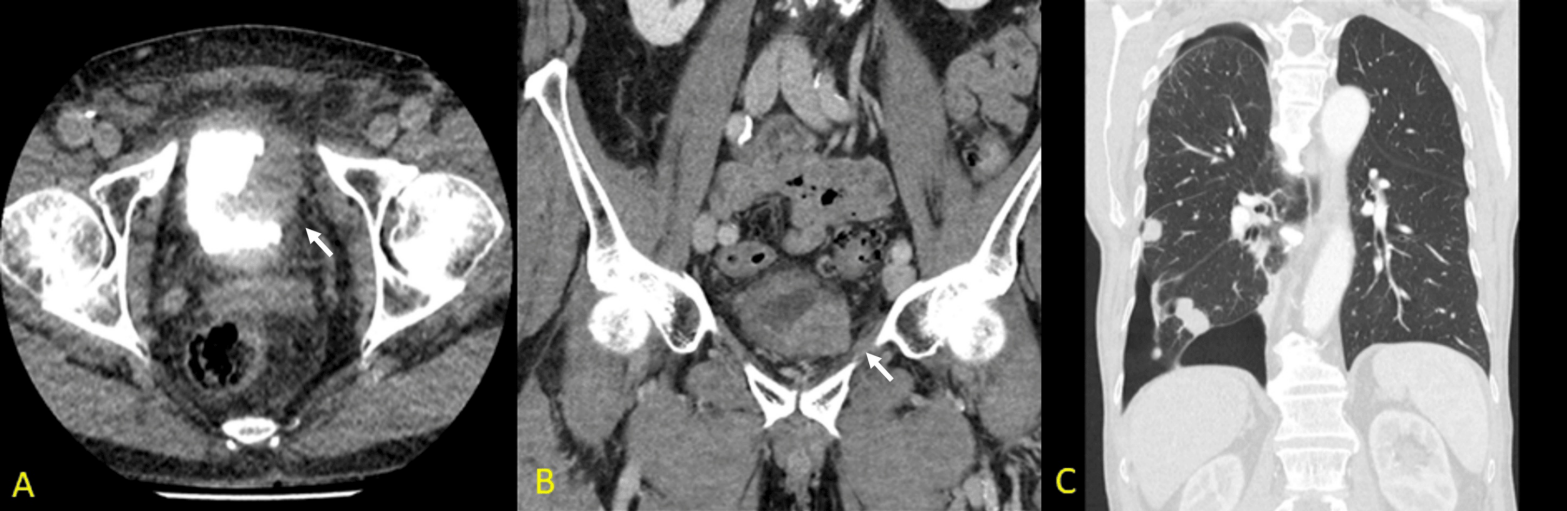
Imaging studies. (A, B) Urinary tract CT revealed an irregular, partial hyperreflective and reflective lesion growing through the bladder (white arrows). (C) A follow-up CT showing pulmonary metastasis.
